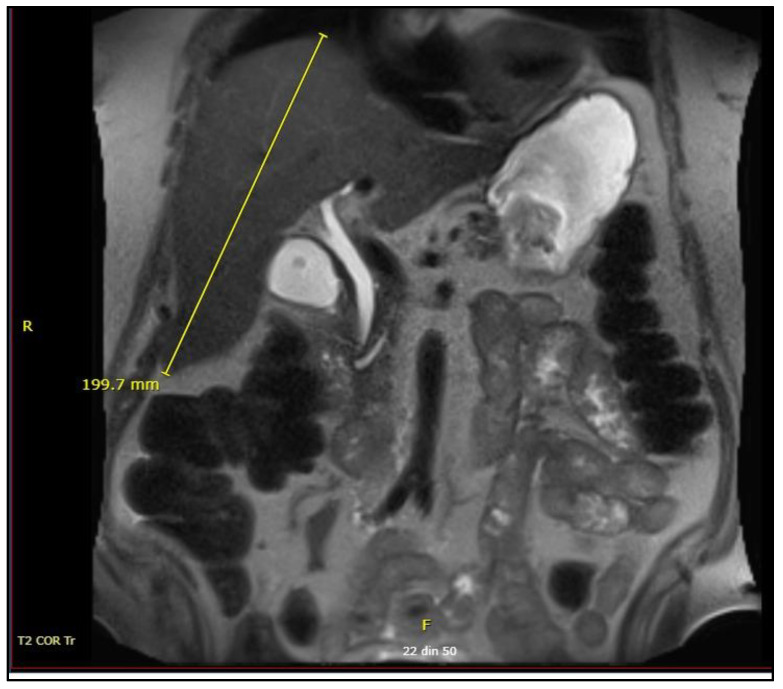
Computed tomography (CT) of the abdomen; R—Right; F—Frontal view.
